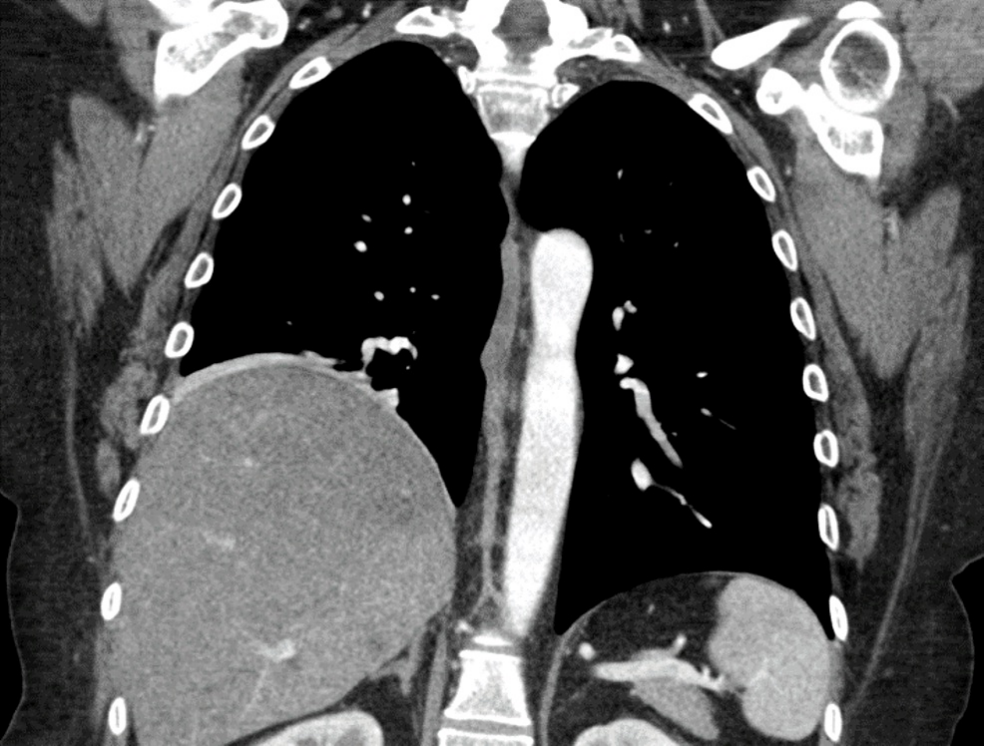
Coronal chest CT showing elevated right hemidiaphragm due to unilateral phrenic nerve palsy.
